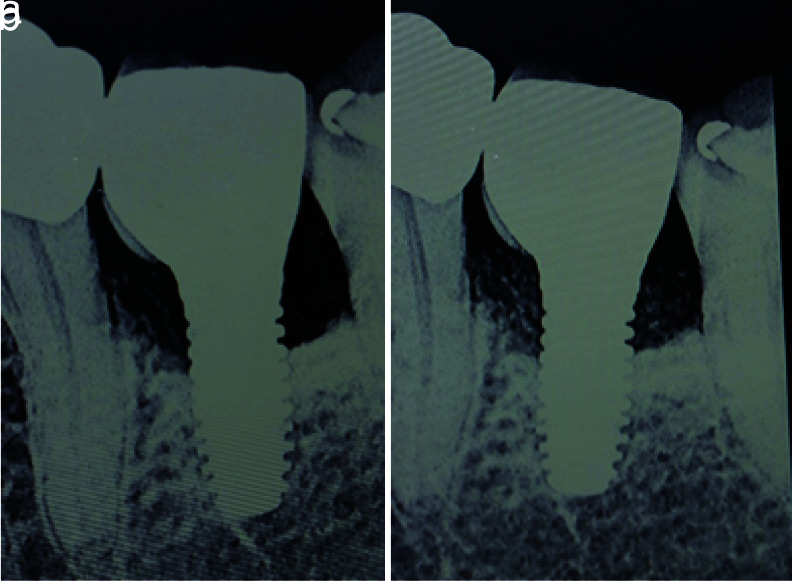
a: Pre-operative radiograph and b post-operative radiograph (taken at 5 months)
